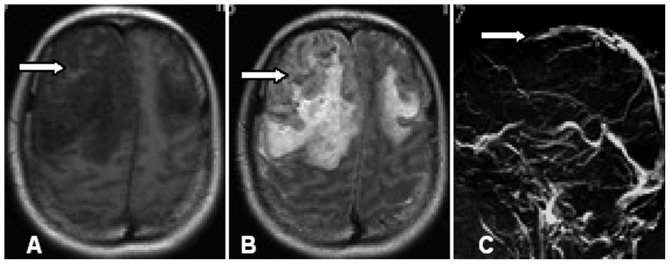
Images of CVT affecting bilateral superior sagittal sinuses by MRI + MRV. (A and B) Long T1 and T2 signals in the bilateral frontal lobes and spot-like slice equal T1 and T2 signals in the focus according to MRI (indicated by the arrows); (C) absence of the blood flow signal at the anterior segment of the superior longitudinal sinus according to MRV (indicated by the arrow).
